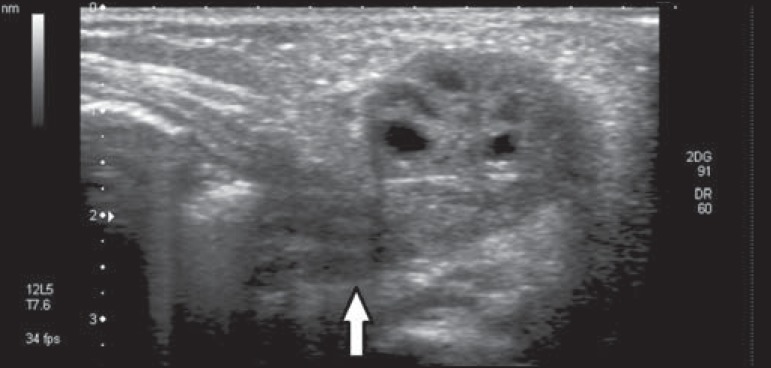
Ultrasound of the right labia majora. Note the invasion of the pedicle
